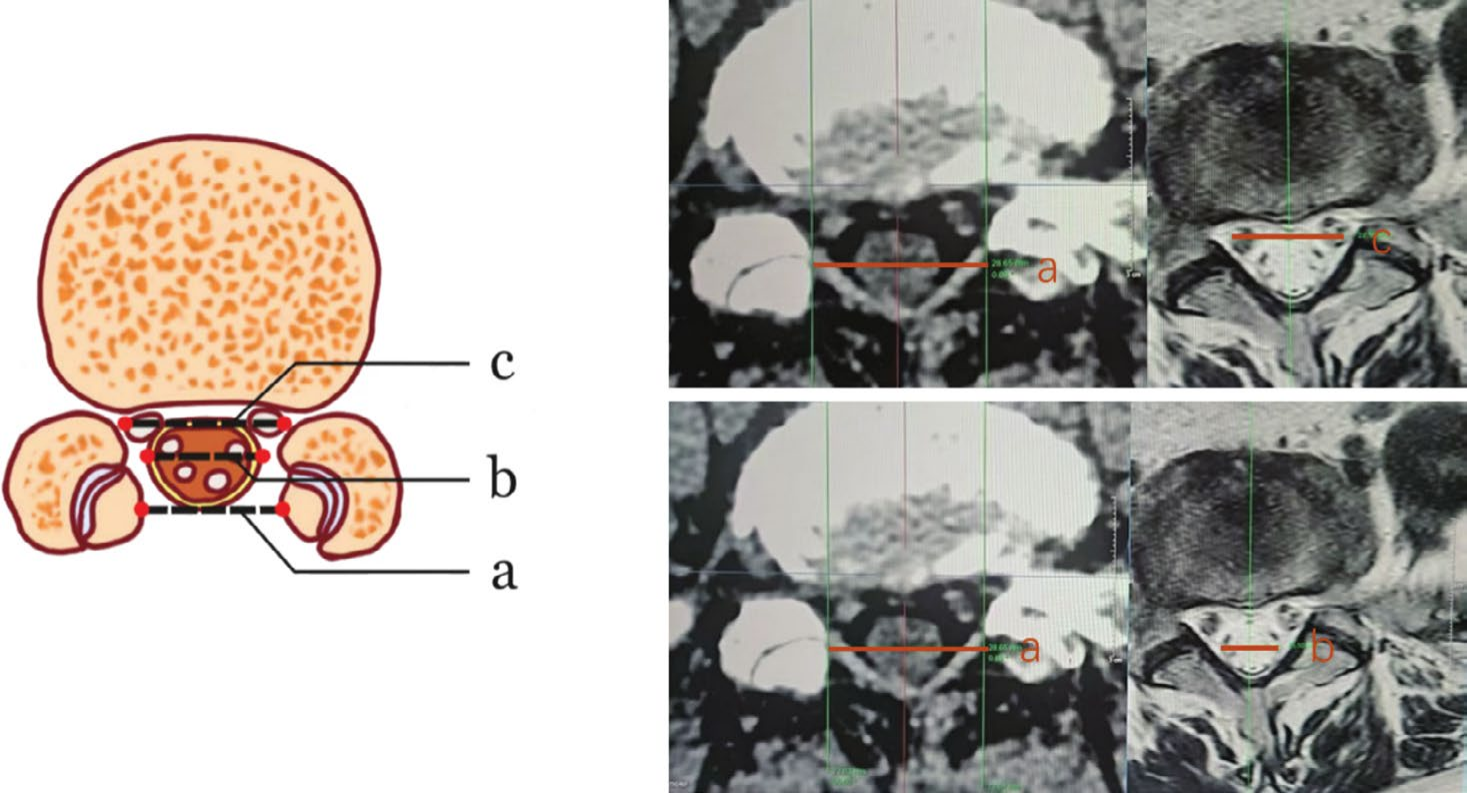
a Refers to the transverse diameter of the intersection point of the two sides (based on CT data), b refers to the transverse diameter of the dura mater at the same level (based on MRI), and c refers to the distance between the outer edges of the S1 nerve at the same level (based on MRI).
